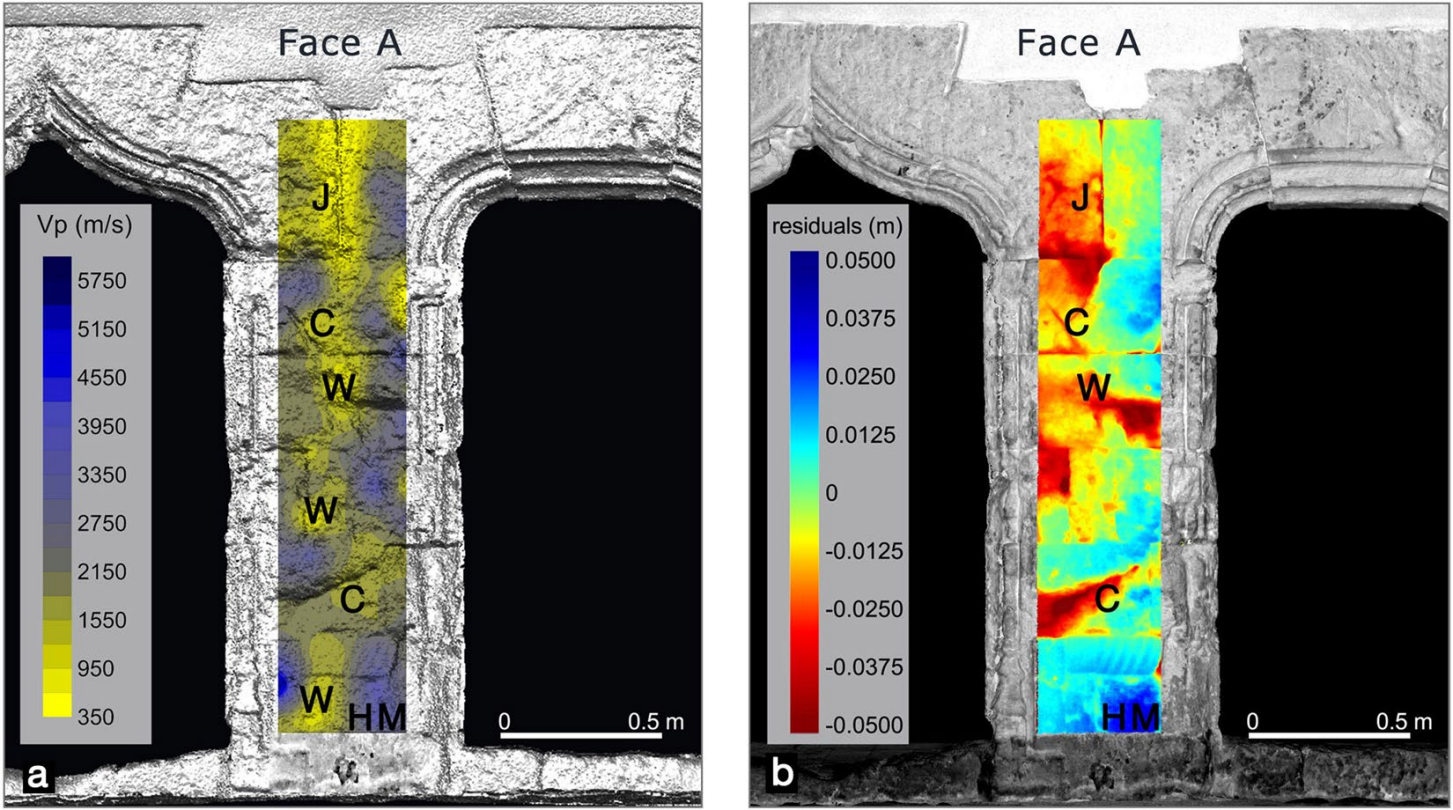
Comparison between the ultrasonic map and the TLS results on face A of the pillar. The two techniques of the NDT survey show matching results highlihted by uppercase letters (C = crack; J = Junction; W = Weathering; HM = Health Material).
